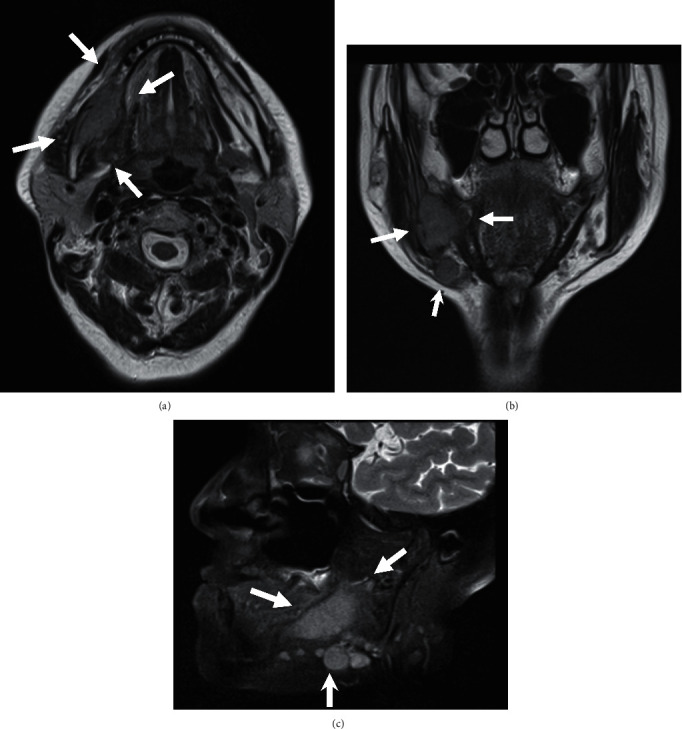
MR image. (a) Axial view. (b) Coronal view. (c) Sagittal view. T1-weighted MR reveals a 45 mm × 2 3 mm × 19 mm low-signal mass in the body of the mandible involving the mandibular canal. The submandibular LN is swollen.
